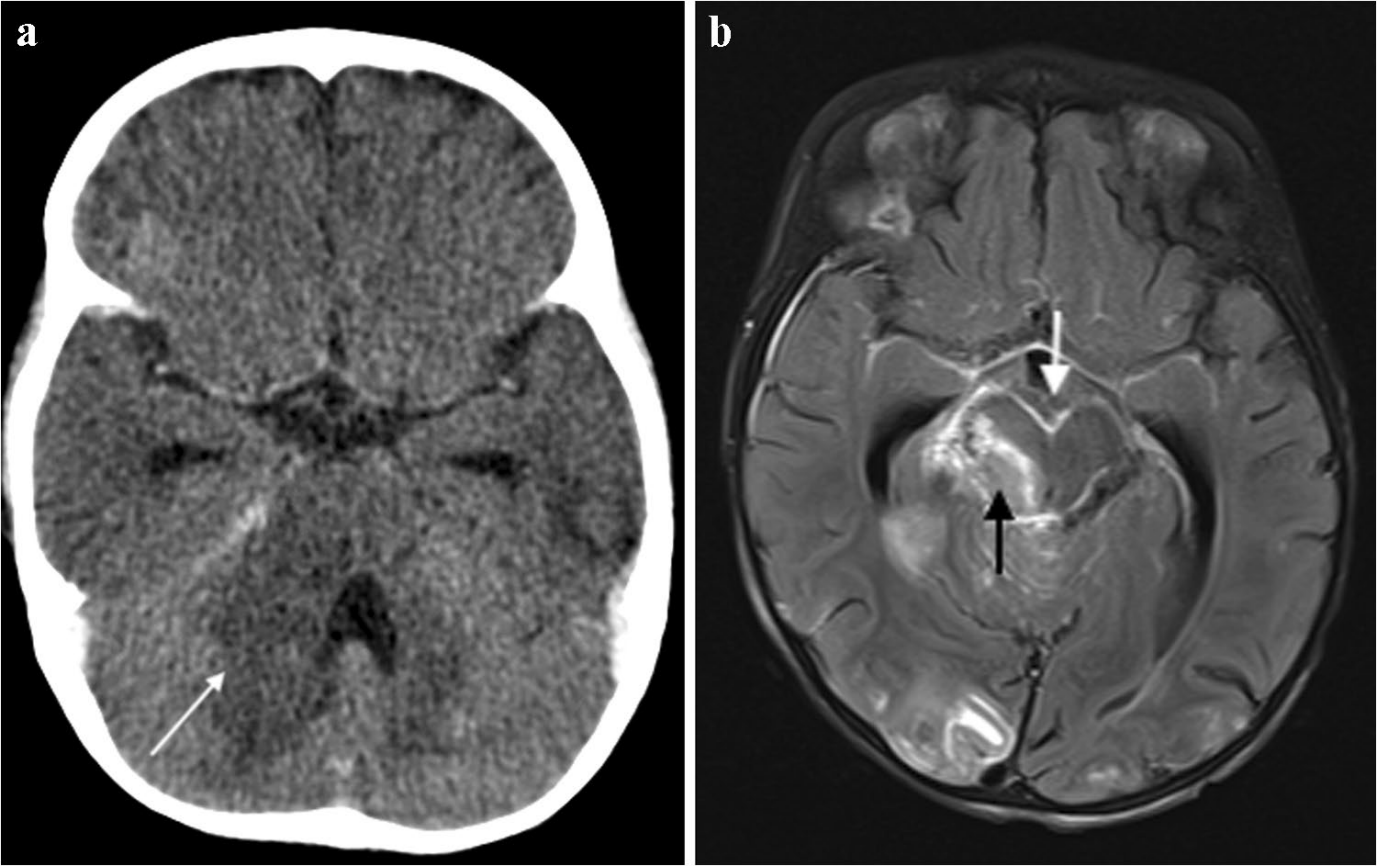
a Brain imaging in an 18-month-old girl with newly diagnosed human immunodeficiency virus infection and presumed tuberculous meningitis. a Axial post-contrast computed tomography shows hypodensity with mass effect in the right cerebellar peduncle (*arrow*), hydrocephalus with temporal horn prominence and absence of basal meningeal enhancement. b Axial T1 post-gadolinium magnetic resonance image clearly demonstrates basal meningeal enhancement, an enhancing mass in the right peduncle (*white arrow*) and multiple tiny miliary nodules (*black arrow*)
